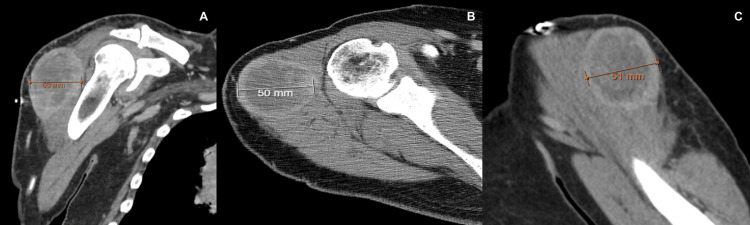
CT shoulder with contrast of R shoulder. (A) The sagittal view with the greatest dimension measuring 50 mm. (B) The axial view with the greatest dimension measuring 50 mm. (C) The coronal view with the greatest dimension measuring 51 mm.
